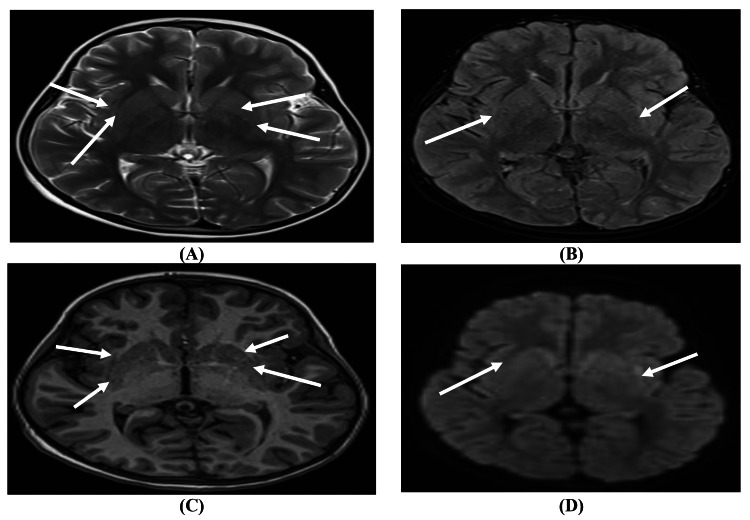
Characteristic findings of autoimmune encephalitis in a seven-year-old girl presenting with acute encephalopathy and recurrent seizures. (A) Axial T2-weighted imaging sequence. (B) Axial FLAIR sequence. (C) Axial T1 magnetization-prepared rapid acquisition gradient-echo sequence. (D) Axial DWI sequence showing bilateral external capsule/claustrum hyperintensities in T2/FLAIR with restriction in DWI T2/FLAIR: T2-weighted/fluid-attenuated inversion recovery; DWI: diffusion-weighted imaging
